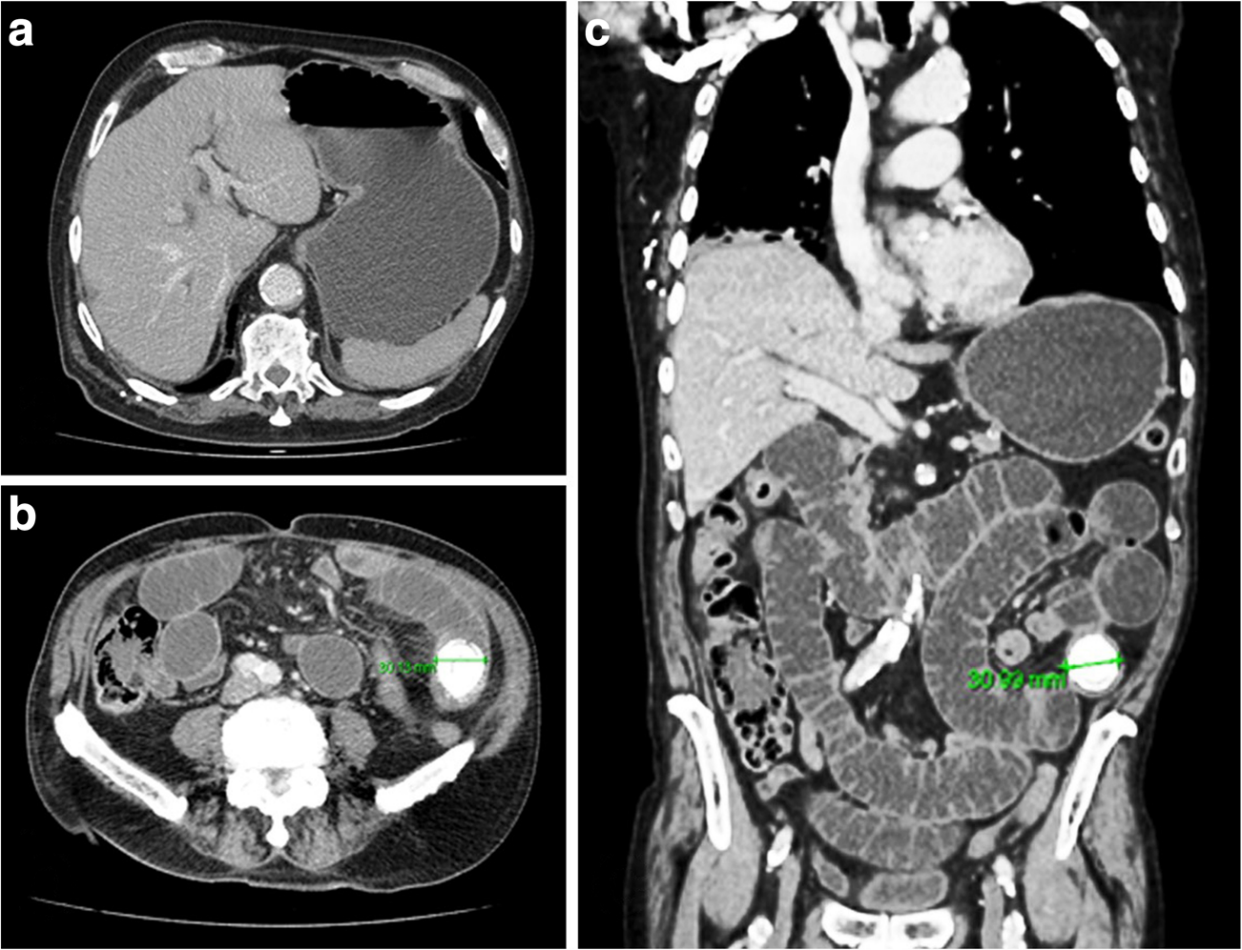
Contrast CT abdomen/pelvis images at time of admission. a CT slice demonstrating a dilated, fluid-filled stomach. Nil significant pneumobilia noted. b, c CT slices demonstrating an obstructing, calcified mid-jejunal intraluminal stone measuring 3 cm in diameter. Evidence of small bowel obstruction noted with dilated jejunal loops above the obstruction. Colon is of normal caliber
